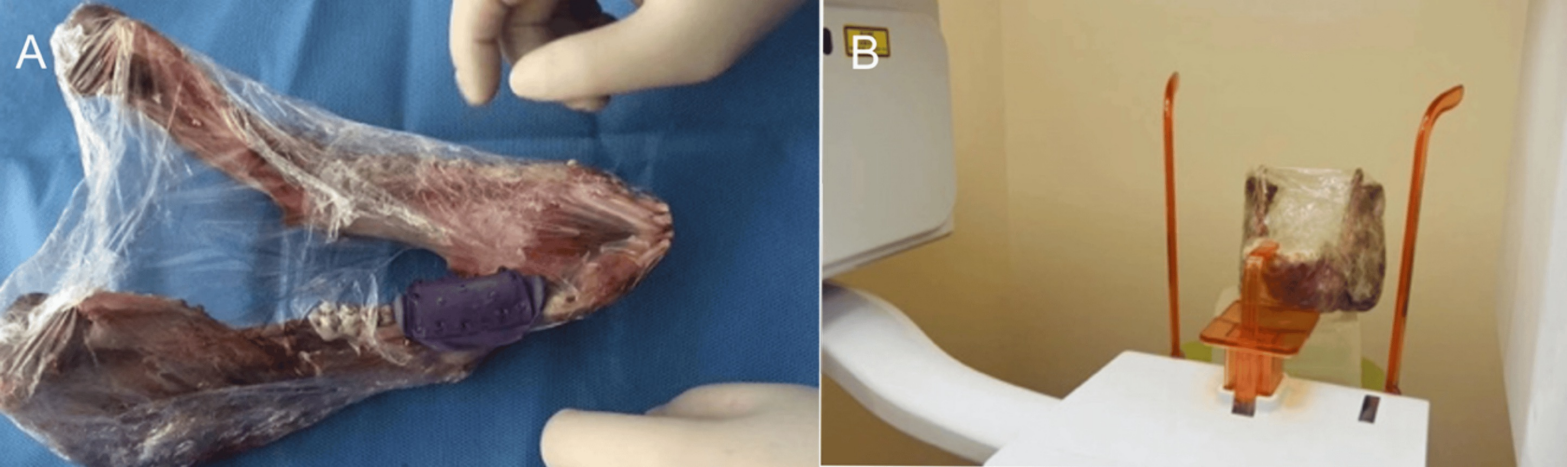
(A) Installation of the registration device. (B) CBCT imaging with the registration device installed CBCT: cone beam computed tomography
